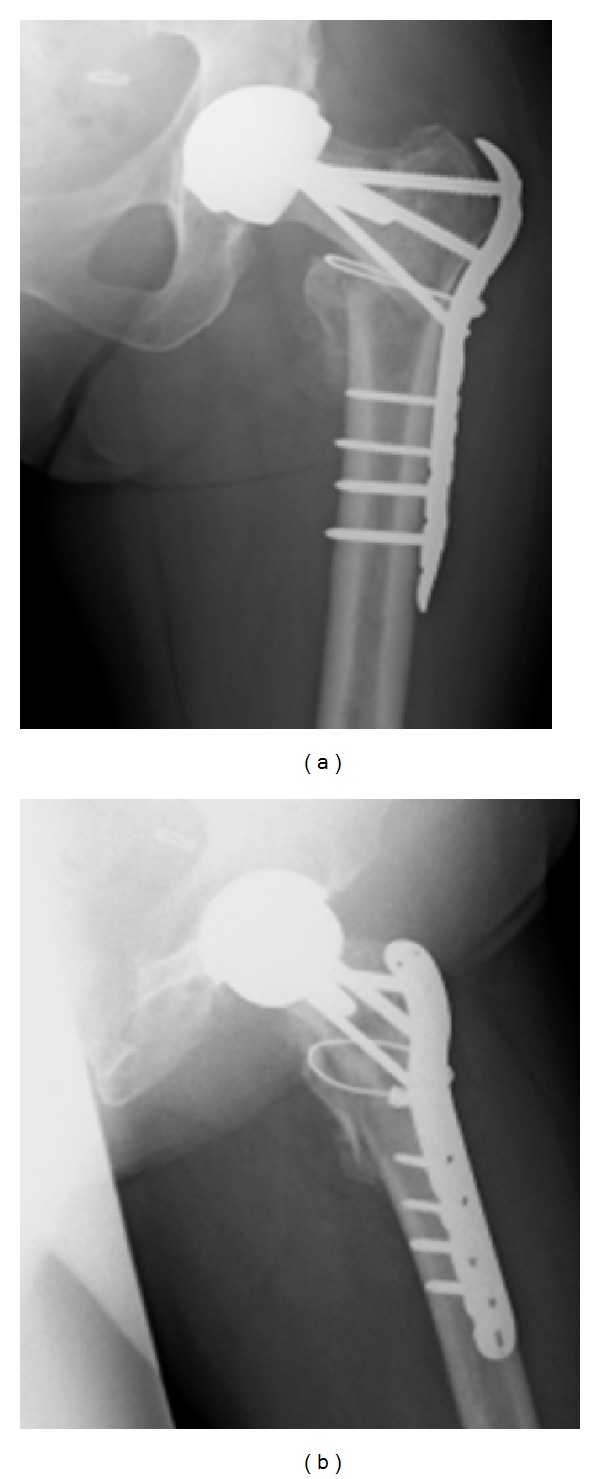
(a)-(b) Radiographs from 12 weeks after operation.
